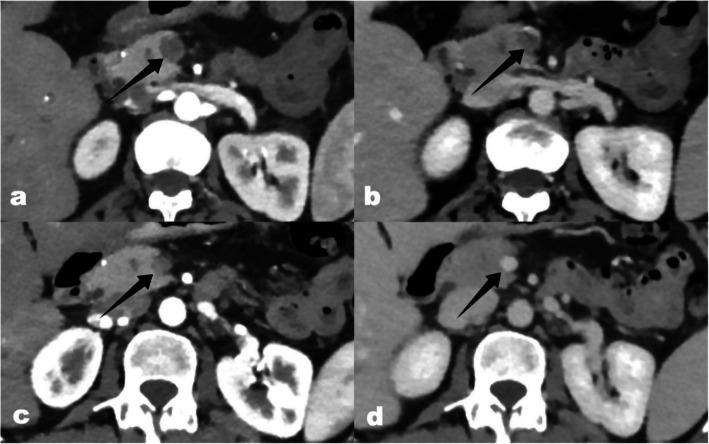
Preoperative enhanced-CT (a) arterial phase and (b) venous phase SMV thrombosis is visible (black arrow); 2 months follow-up enhanced-CT (c) arterial phase and (d) venous phase complete removal SMV thrombus (black arrow); SMV superior mesenteric vein
